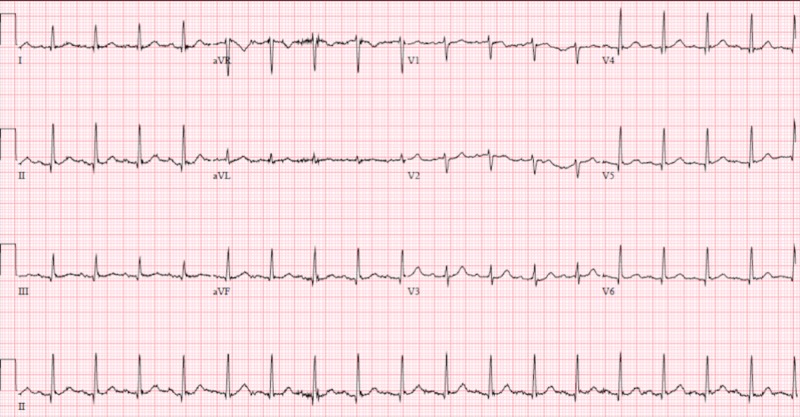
Baseline 12-lead echocardiogram from admission
